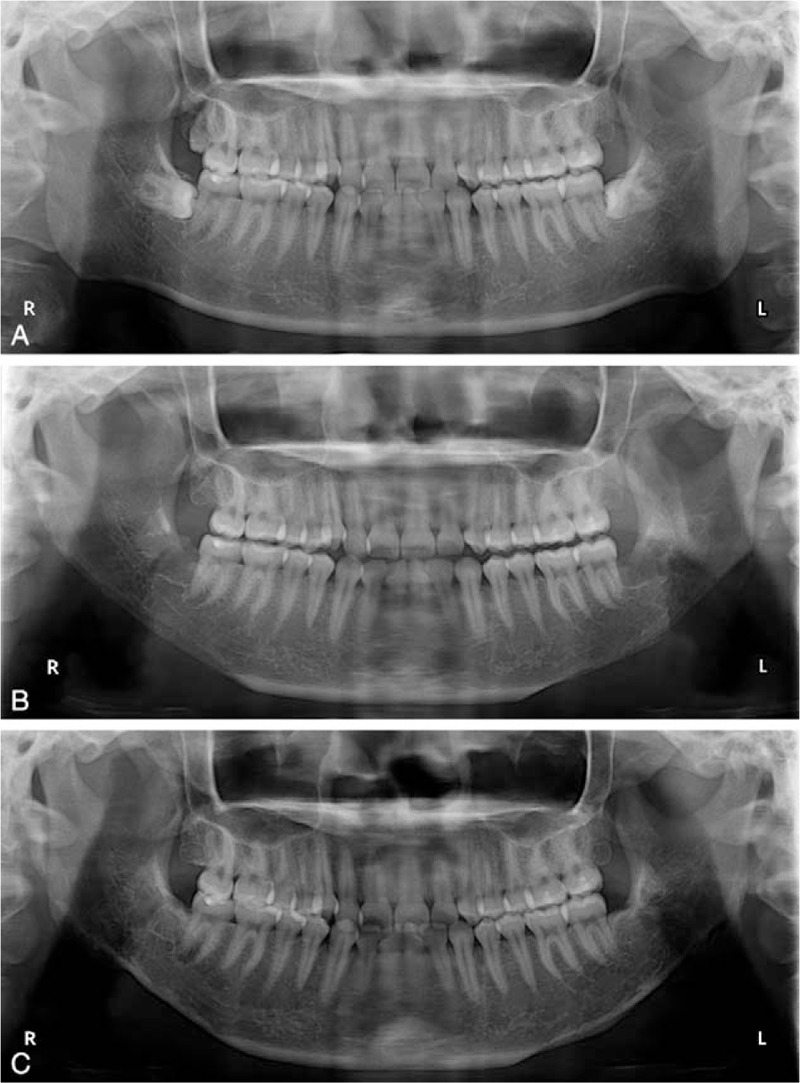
Mandibular panoramic radiographs of patient 2. The pre-op radiograph showed not only the bulged mandibular rami and body, but also the impacted wisdom teeth and right maxillary third molar (A). The short-term post-op radiograph showed that the impacted molars were completely removed without fracture line, and that the ostectomy lines were naturally curved (B). Six months after surgery, the bone healed completely (C).
